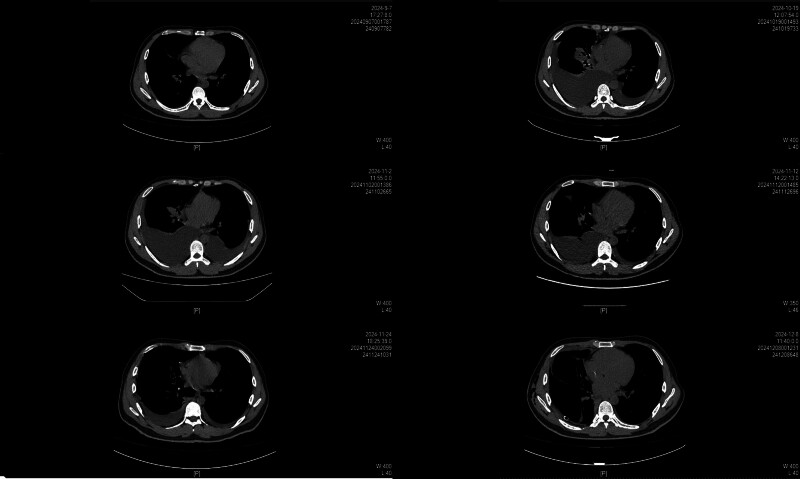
Chest CT scans showing pleural effusion before surgery in different periods and few of them after surgery. CT = computed tomography.
